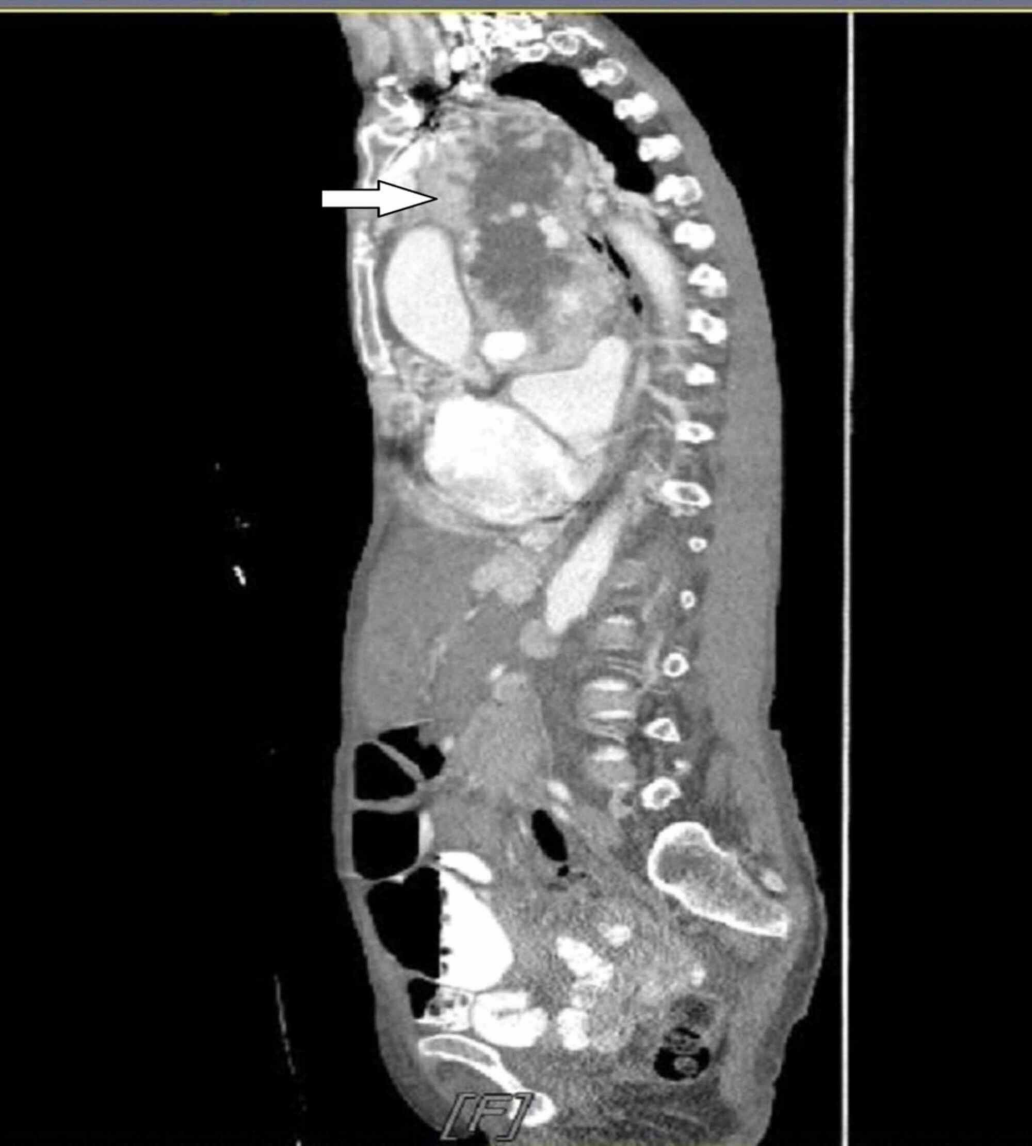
CT scan: sagittal section of the chest and abdomen revealing a large upper anterior mediastinal mass with central necrosis and with a mass effect on the heart
